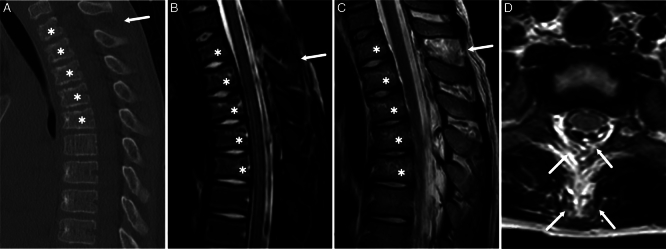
A 12-year-old boy after a trampoline accident. Complete posterior ligamentous complex injury (arrows) in a level Th1/Th2 and compression fractures on vertebrae Th1, Th2, Th3, Th4, and Th5 (asterisks). (A) Noncontrast computed tomography, sagittal plane, (B) Magnetic resonance imaging (MRI), short tau inversion recovery, sagittal plane, (C) MRI, T2-weighted, sagittal plane, and (D) MRI, T1-weighted, axial plane.
